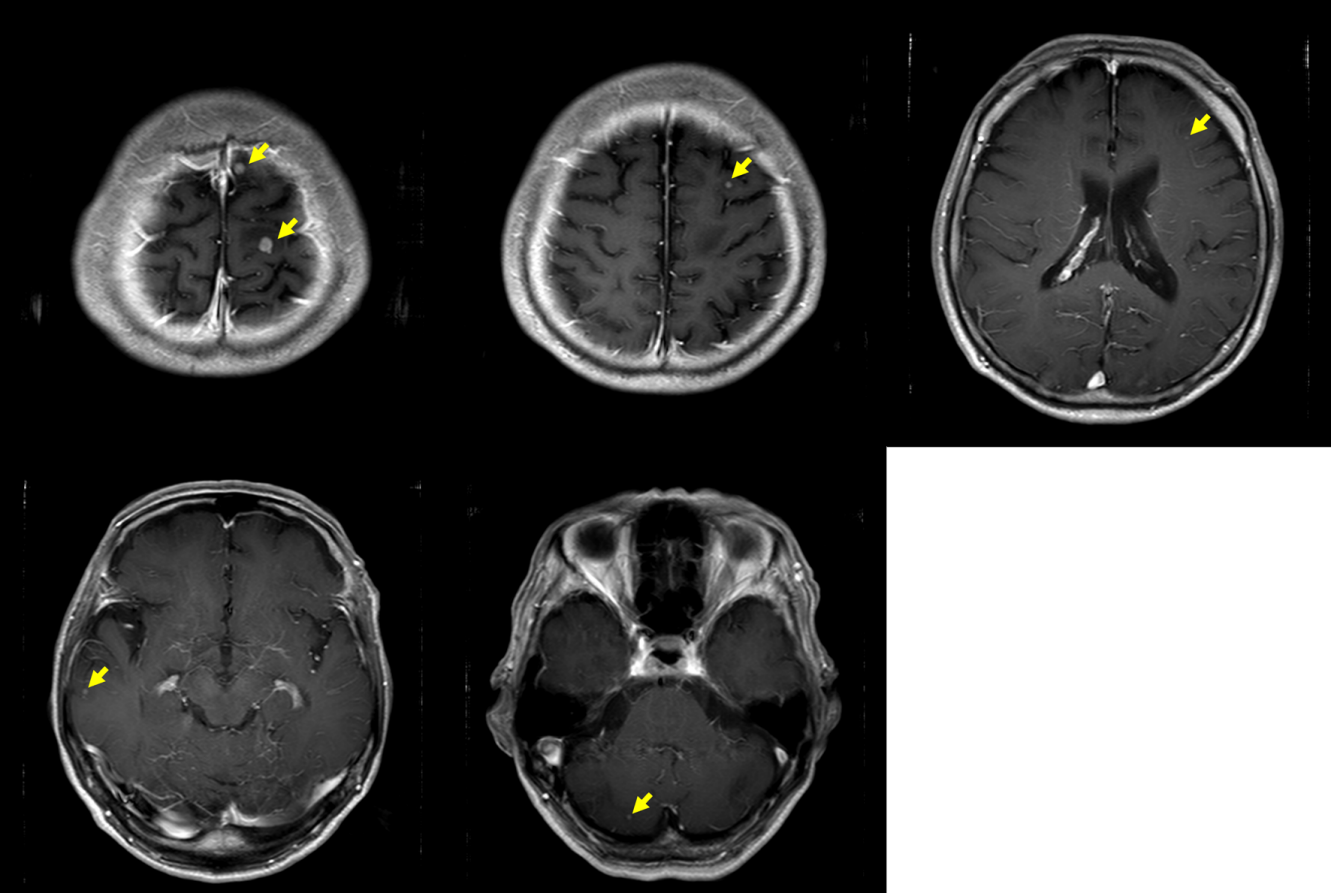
Magnetic resonance imaging showed multiple brain metastases (yellow arrows) but no significant neurological symptoms
